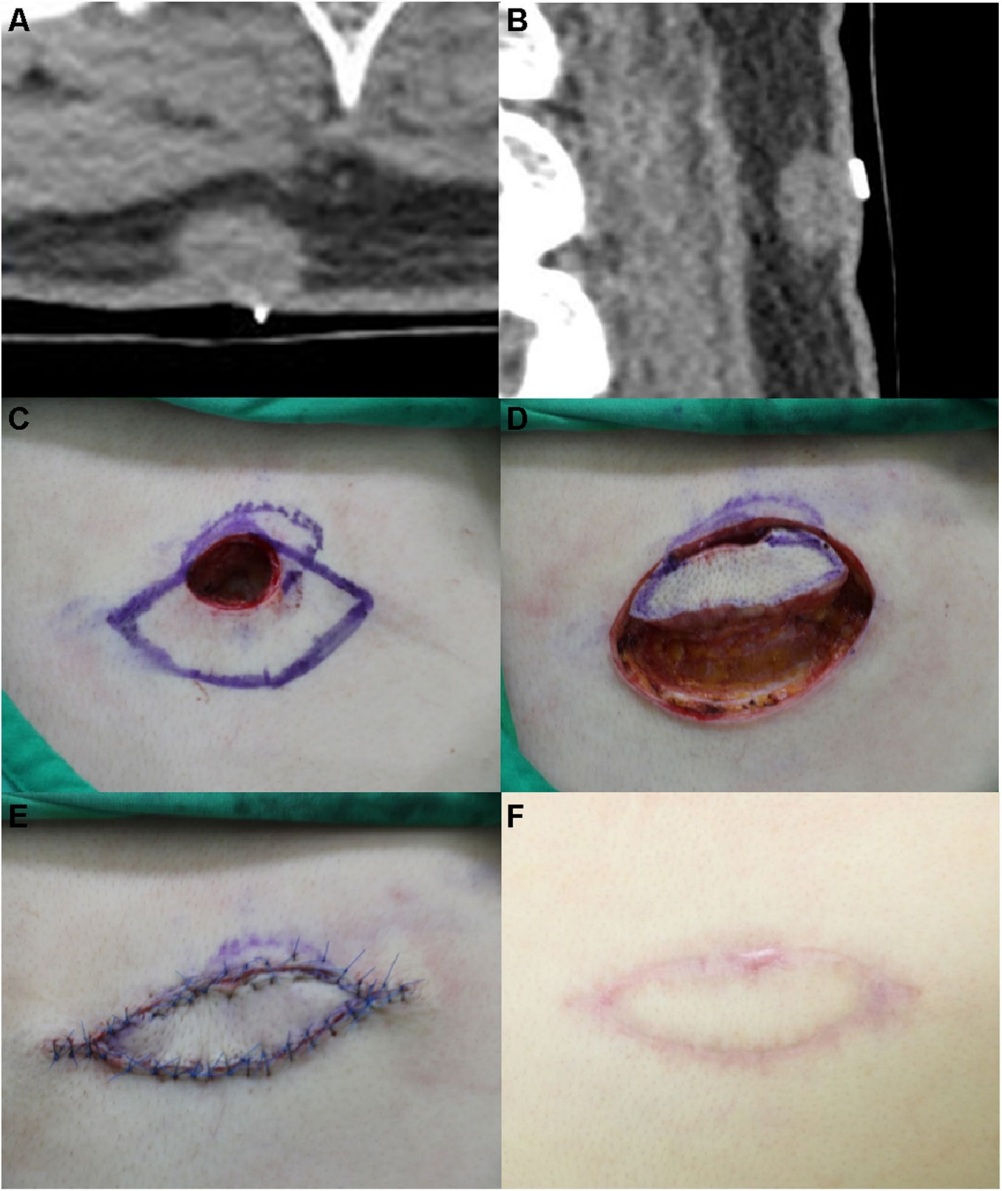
Keystone-design perforator island flap (KDPIF) reconstruction after complete excision of the ruptured epidermoid cyst (EC) in a 36-year-old woman. (A,B) Computed tomography scan revealed a 1.5 × 2 cm elliptical cystic lesion extending from the skin to the deep fat layer at the level of thoracic inlet. (C) The design of the flap. The final defect size was 2 × 2.5 cm and a 2 × 5 cm-sized KDPIF was designed in the lower side of the defect. (D) The island-form of the flap was designed and the flap was moved to the defect with minimal tension. (E) Postoperative clinical photograph immediately after the flap inset. (F) Postoperative clinical photograph of the flap after 10 months of follow-up.
